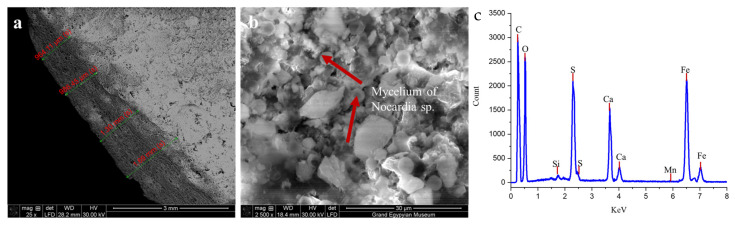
(a) shows the thickness of the formed biofilm, which ranged from 964 µm to 1.69 mm, while the mycelium of *Nocardia* sp. is clearly detected in image (b), as indicated by the red arrows. The panel (c) image shows the elemental composition of a biologically infected sample using energy-dispersive X-ray analysis (EDX).
